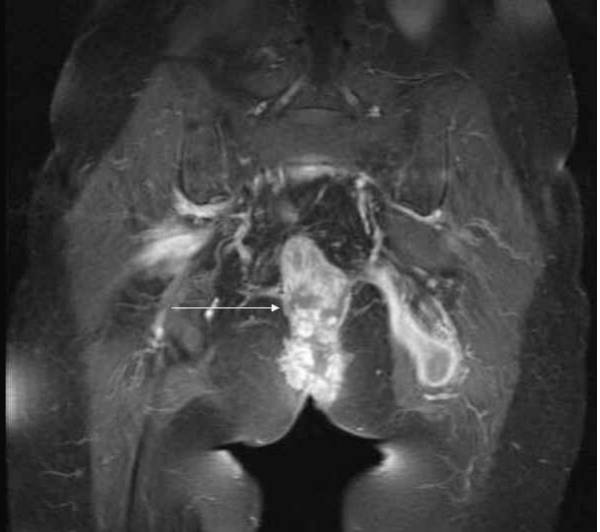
T1- weighted fat saturated MR image after i.v. application of contrast medium coronal. The arrow is pointing to a fistulating formation spreading in the gluteal muscles on the left side with typical rim enhancement and central liquid components; the soft tissue mass seen in midline has a centrally necrotizing appearance.
